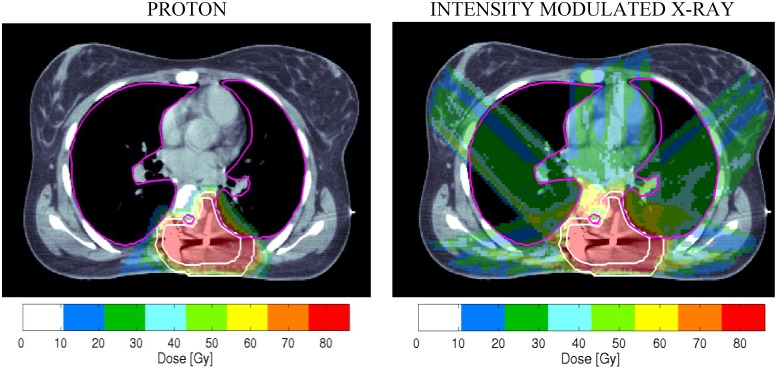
Some clinical dilemmas in particle therapy are shown in these dose distributions displayed on a CT scan of a female patient who has had incomplete surgery for a paravertebral epithelioid sarcoma. (Plan obtained with permission of J. Munzenrider, Massachusetts General Hospital).
